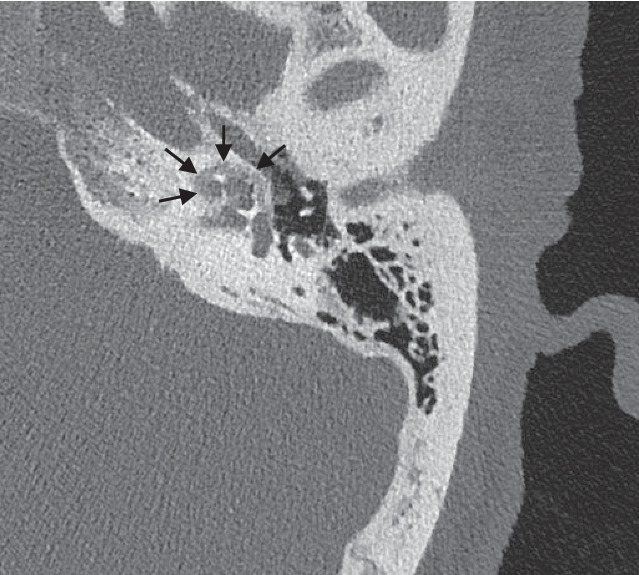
In der Literatur sind bisher mehrere Grading-Systeme zur Einteilung der Otosklerose in der CT beschrieben, jedoch konnte sich keines davon weitgehend durchsetzen. Einzig das Grading-System nach Symons und Fanning [16] wurde häufiger zitiert als andere, wobei eine sehr gute Übereinstimmung zwischen den Untersuchern sowie auch bei mehrmaliger Beurteilung durch einen einzelnen Untersucher gezeigt werden konnte [13]. Weitere Vorschläge zur Klassifikation der Otosklerose in der CT wurden unter anderem durch Veillon et al. [30] und Dudau et al. [4] beschrieben. Das Grading nach Veillon ist hauptsächlich in Frankreich verbreitet und teilt die Otosklerose nach Lokalisation in vier Typen ein. Dudau et al. beschrieben im Jahr 2017 eine Einteilung der Otosklerose, welche auch klinisch relevante Lokalisationen wie das runde Fenster oder die Beteiligung des cochleären Endosts einschließt.
